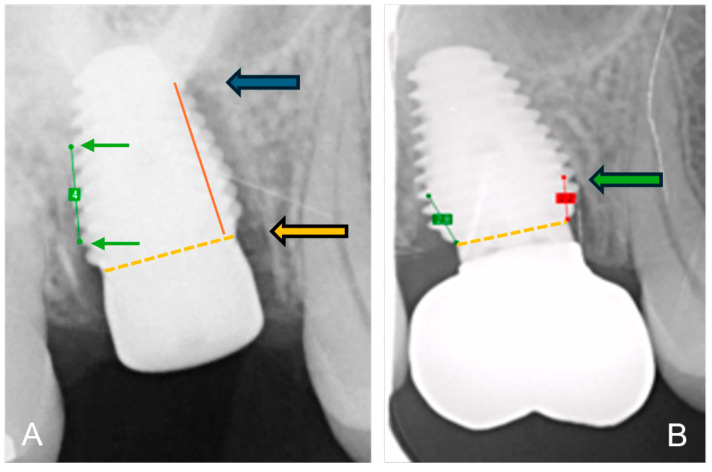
Method of radiographical assessment of an immediately placed MAX implant in a molar. Extraction socket; (A) after surgery: green line is the calibrated measurement of 5 implant pitch threads corresponding to 4 mm in reality; the orange dotted line indicates the implant-abutment interface and the orange arrow indicates the reference point; the red line the distance from bone level to reference point and the blue arrow indicates bone level at baseline immediately after surgery; (B) at final recall: the green arrow indicates the bone level at final recall; the red line the distance from bone level to reference point.
